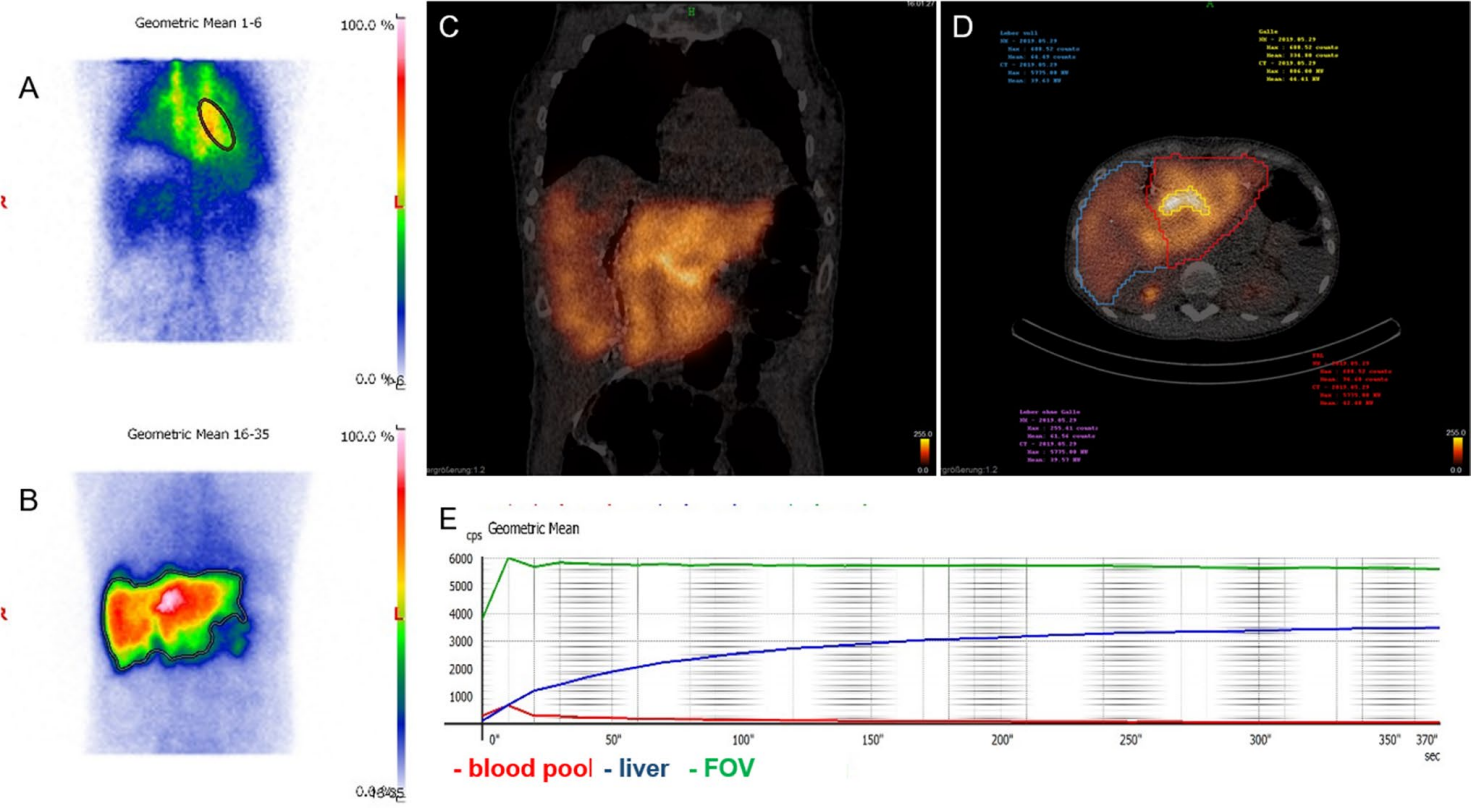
Clinical example of a patient (female, 56 years) with hepatic metastasis of colorectal cancer. A Geometric mean of the early frames of the first dynamic sequence depicting the ROI of the left ventricle. B Geometric mean image of the first dynamic sequence (150–350 s) with ROI of the total liver. C Coronal slice of the SPECT CT fusion image depicting the mebrofenin uptake in the liver after ALPPS-procedure. D Transversal slice of the fused SPECT and CT after segmentation of the total liver, FRL, and intrahepatic bile ducts. E Time-activity curves of the first dynamic scan for blood pool, liver, and whole field-of-view used for calculation of MUR
